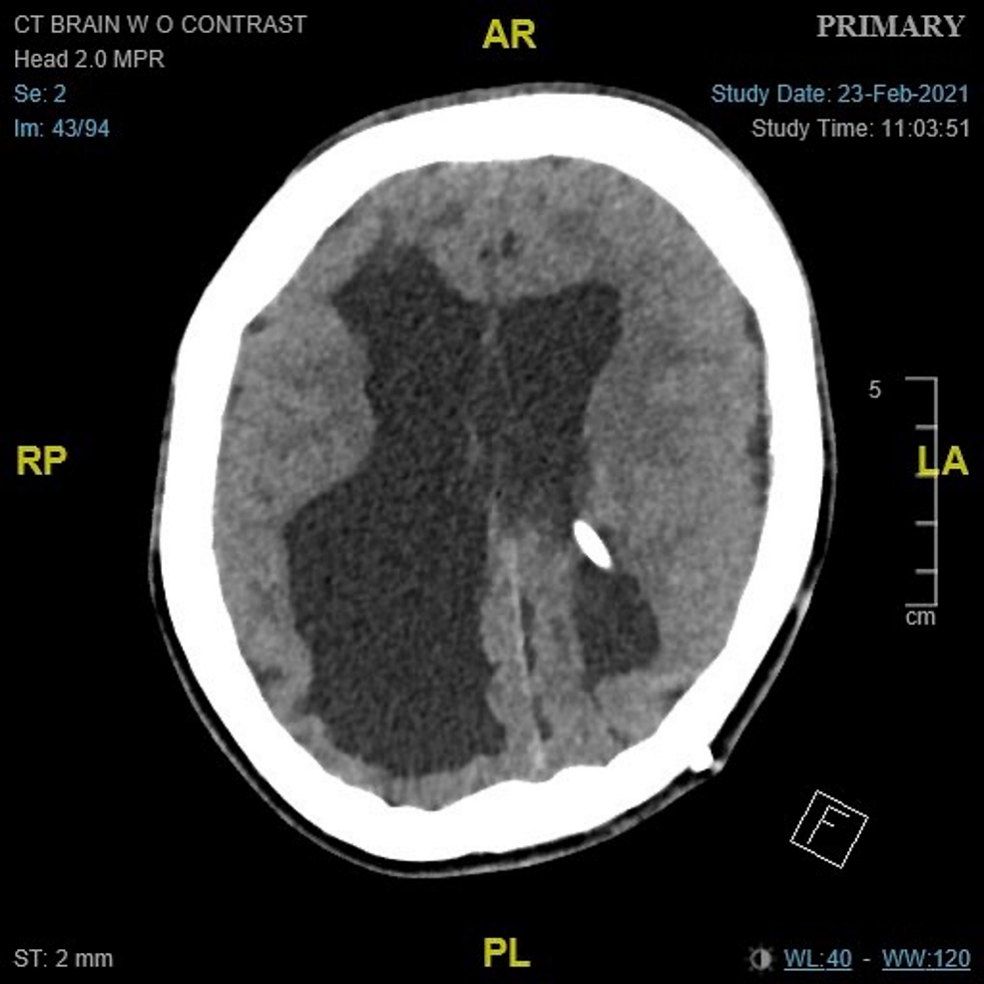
CT brain axial cut at the time of presentation in ER shows ventricular dilatation and periventricular lucency.
